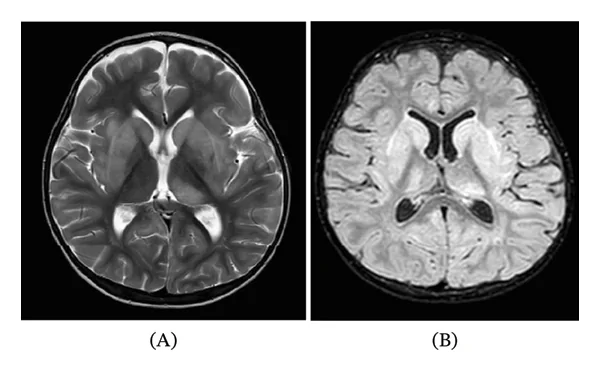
Brain MRI for the patient at the initial presentation: (A) T2 weighted image at the level of the basal ganglia shows bilateral hyperintensity signal involving caudate, putamen, external capsule, and left thalamic. (B) FLAIR axial image at the level of basal ganglia showed the same findings.
